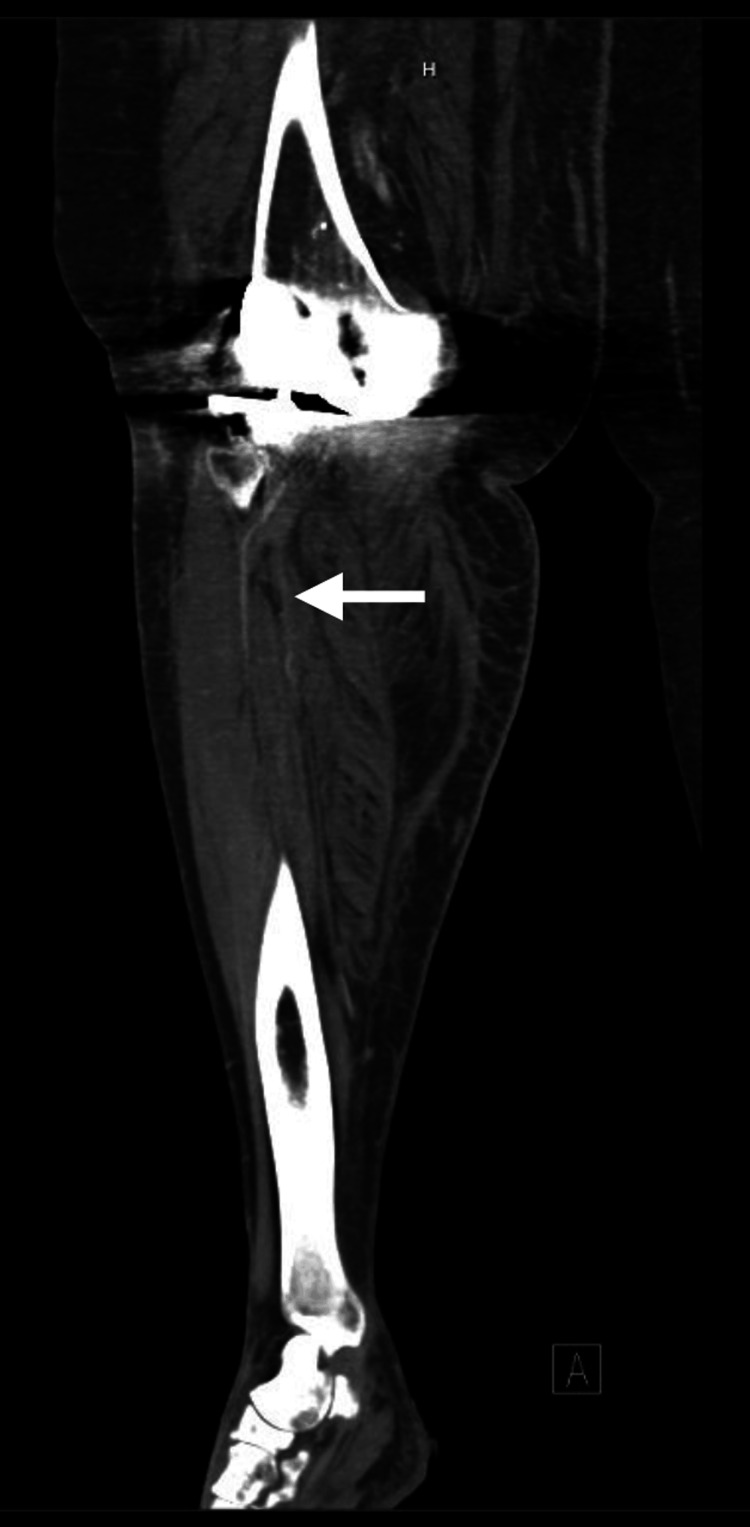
CT angiography (CTA) showing no contrast in the runoff vessels (white arrow), indicating arterial injury.
